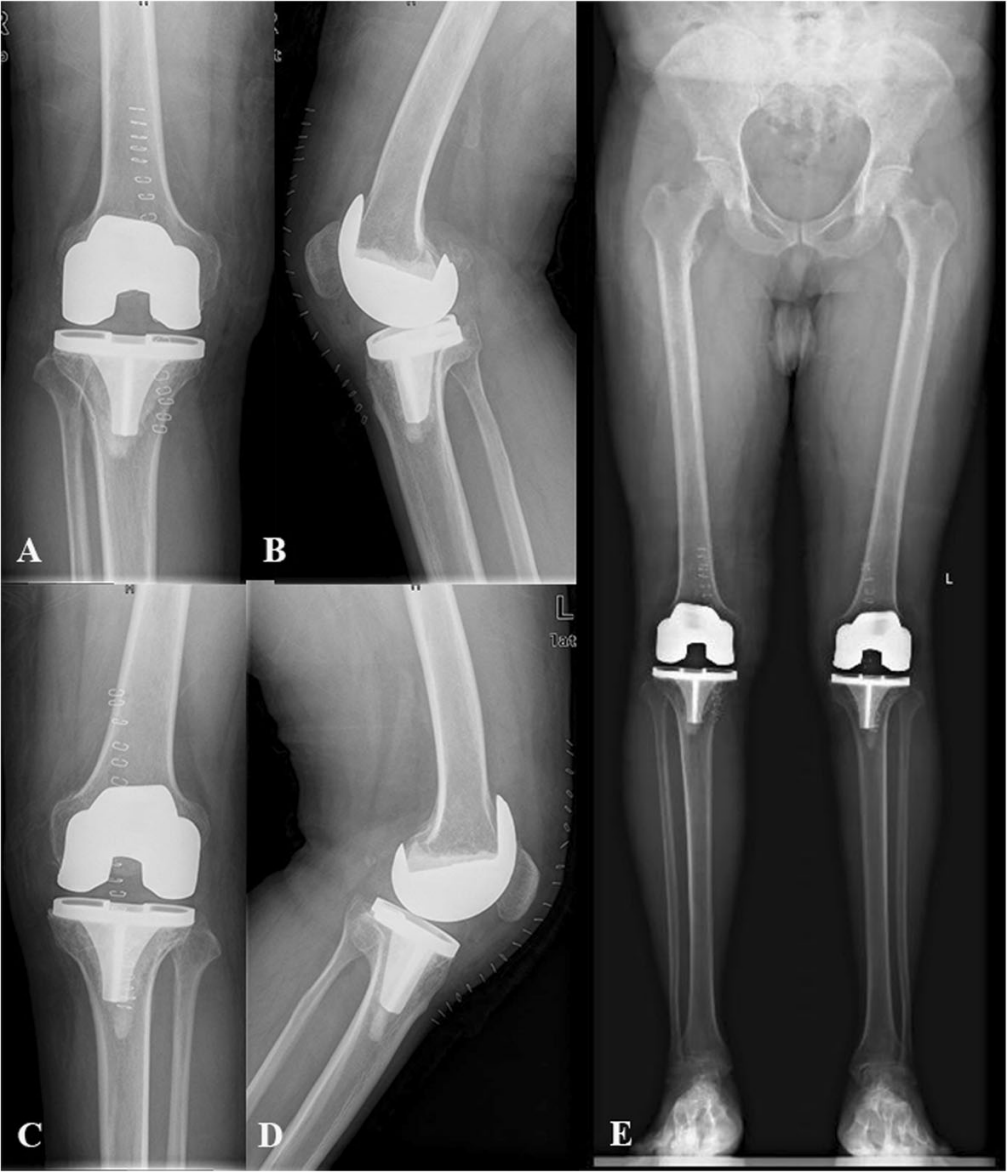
Postoperative X-ray of simultaneous bilateral TKA. a AP view of right knee; b Lateral view of right knee; c Anteroposterior (AP) view of left knee; d Lateral view of left knee; e Full-length standing AP view
